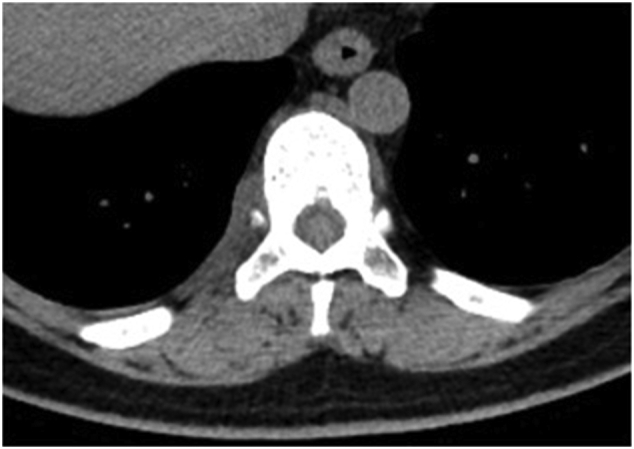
Chest computed tomography did not show fluid accumulation in the cyst 3 months after surgery.
